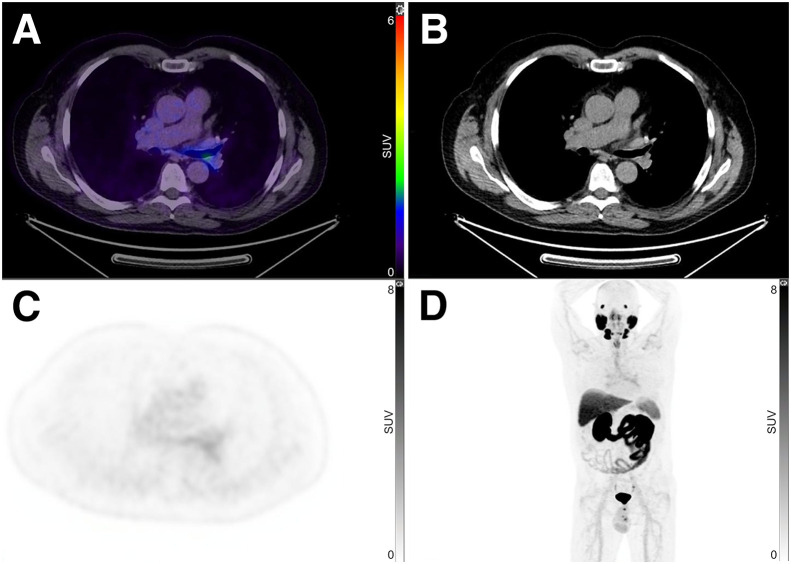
^18^F-DCFPyL fused axial PET/CT (A), CT (B), PET (C), and PET maximum-intensity projection (D) showing tracheal uptake.
